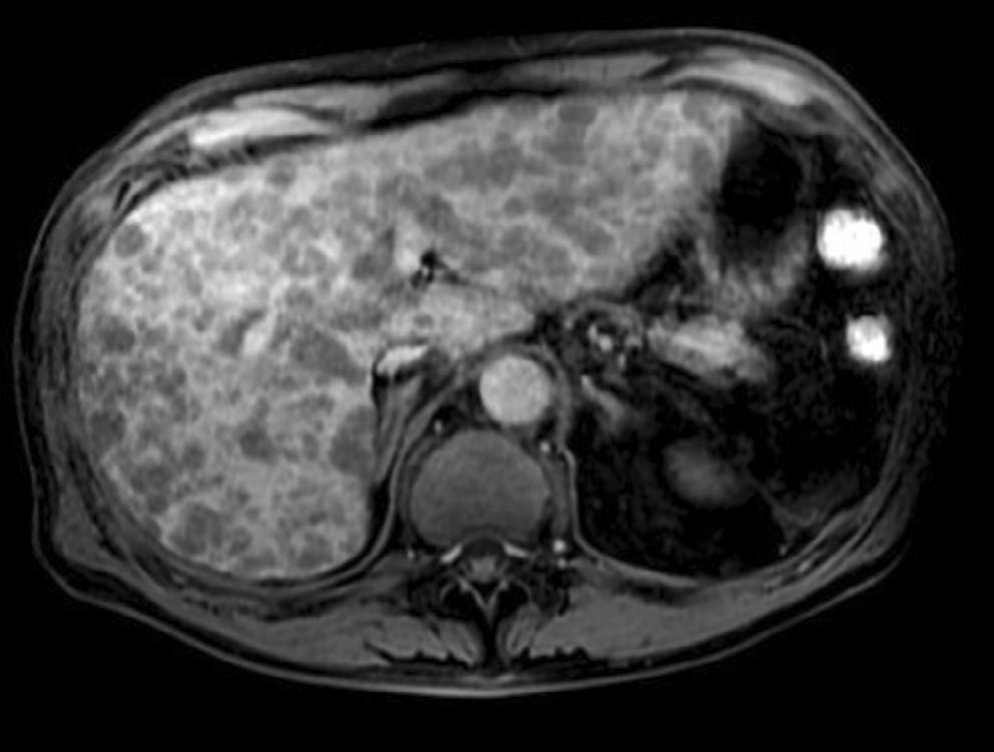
Contrast‐enhanced magnetic resonance imaging in the hepatobiliary phase demonstrates numerous small‐to‐medium‐sized, well‐defined hypointense nodules diffusely distributed throughout both hepatic lobes, without formation of a dominant mass. This multifocal distribution pattern is less suggestive of primary hepatic carcinoma and is more consistent with diffuse hepatic involvement by malignant lymphoma, while diffuse‐type metastatic liver tumors are also considered in the differential diagnosis.
